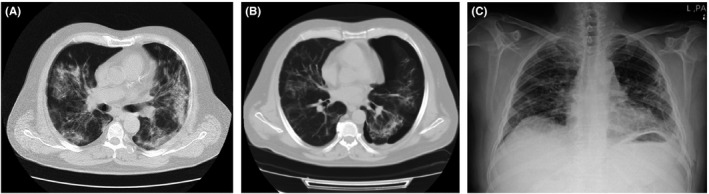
Chest CT scans and chest X‐ray of case 2
